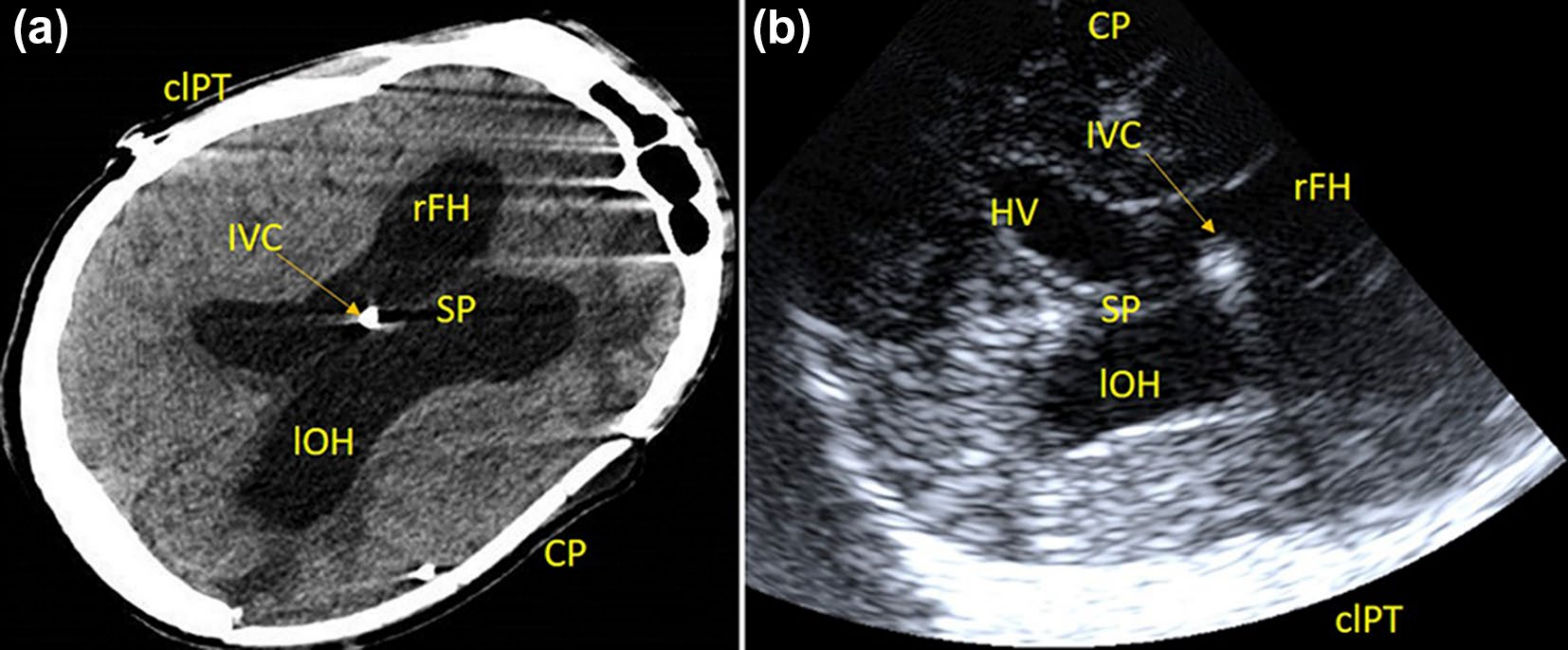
Intracranial evaluation before shunting (a, CT scan; b, transcranial ultrasound). Ventricular size and intracranial anatomy are clearly visible with transcranial ultrasound. In particular, the PEEK window allows us to also identify complications such as hemoventricle. clPT, contralateral parietal teca, CP, cranioplasty, CT, computed tomography, IVC, intraventricular catheter, lOH, left occipital horn, PEEK, polyetheretherketone, rFH, right frontal horn, SP, septum pellucidum
